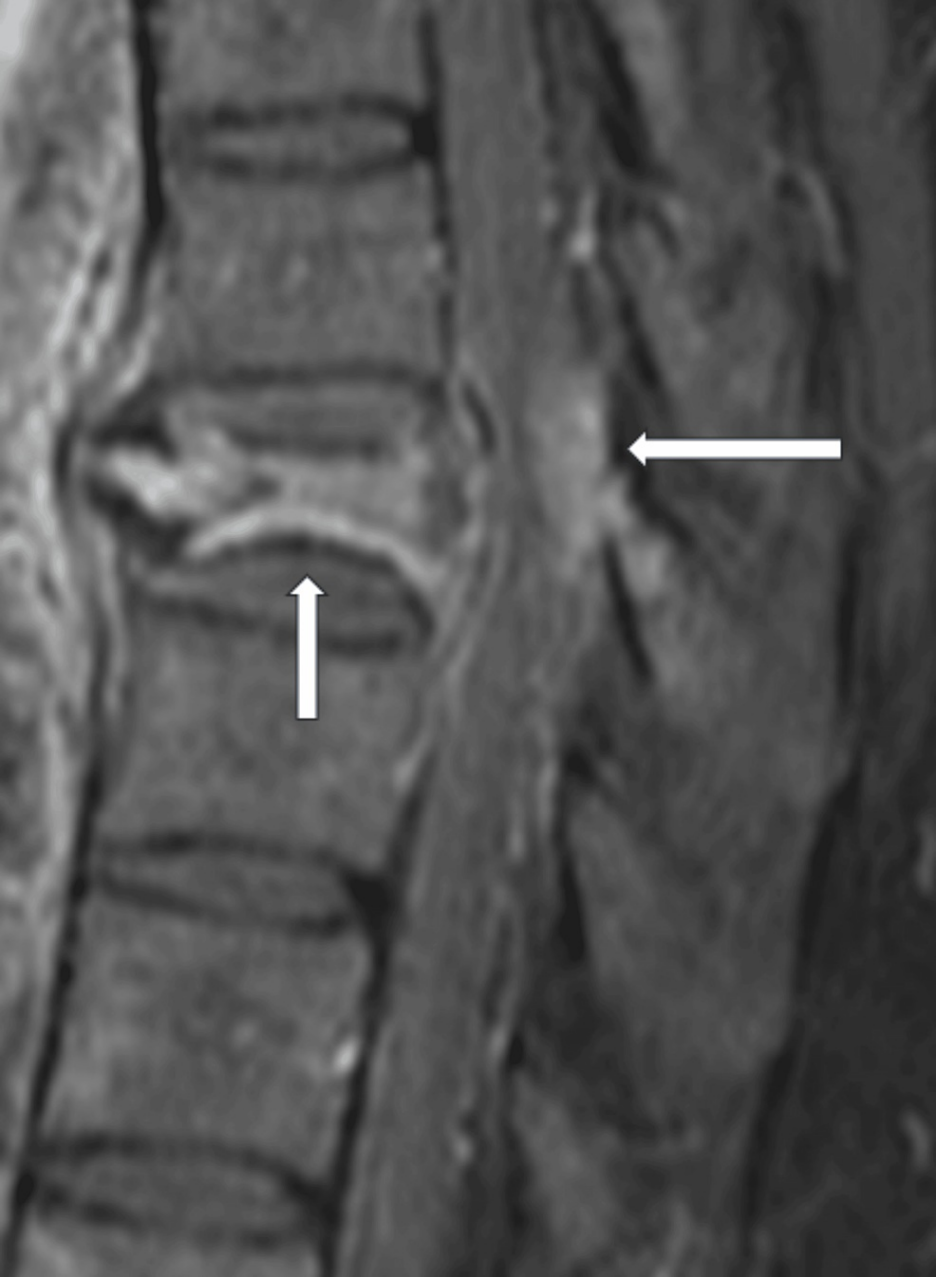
T1- Post-gadolinium contrast - magnetic resonance imaging (MRI), mid-sagittal view of the spine demonstrating a heterogenous contrast-enhancing lesion involving the body with collapse (vertical arrow) with significant spinal cord compression (horizontal arrow)
